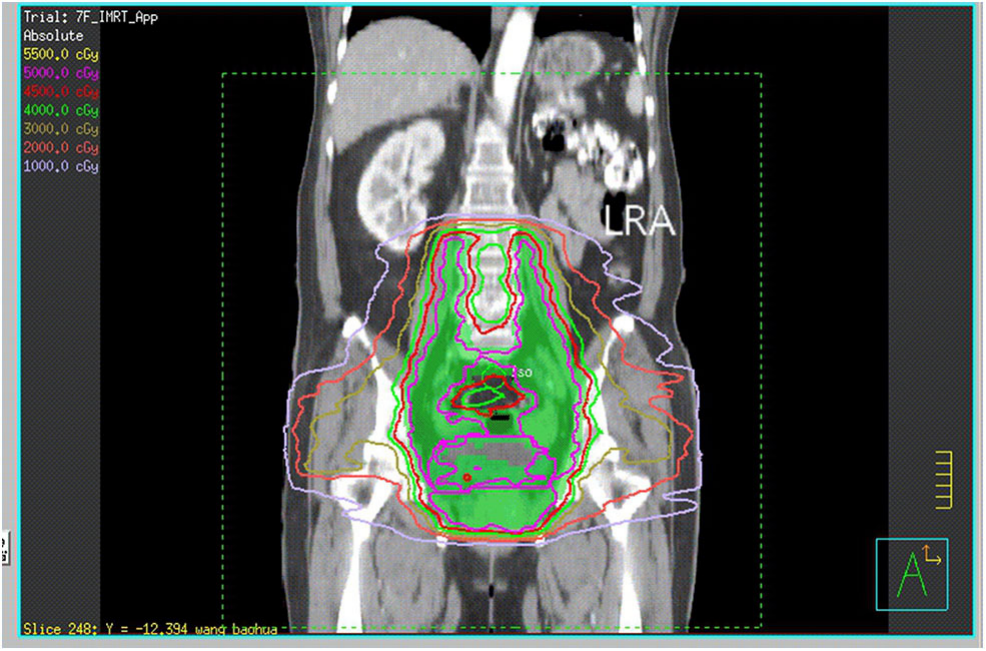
CT reconstruction of the coronal plane shows the upper and caudal field borders. LRA, left renal agenesis.
